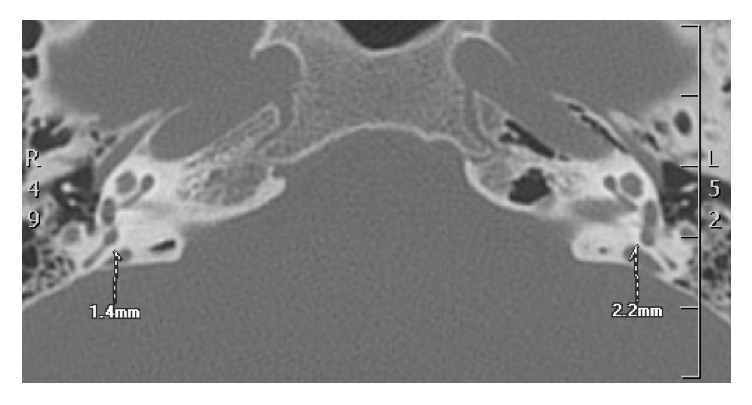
CT scan results: the endolymphatic duct and sac in the left side are larger (2.2 mm) than in the right side (1.4 mm).
